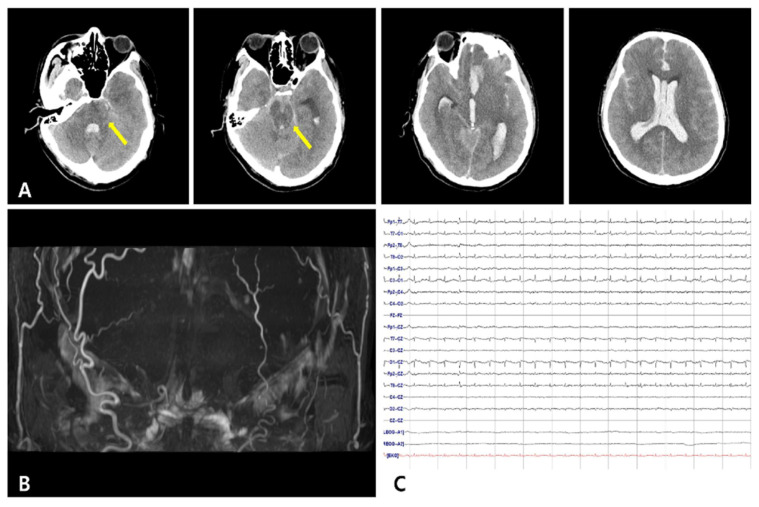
Radiologic findings and electroencephalography of the patient. (A) Brain computed tomography shows intracerebral hemorrhage in the left frontal lobe and massive intraventricular hemorrhage with subarachnoid hemorrhage including the basal cistern. Hypodense lesions in the brain stem were observed due to hypoxic brain damage (arrow). (B) Brain magnetic resonance angiography of the patient shows no cerebral blood flow. (C) There is no identifiable cerebral activity in any lead throughout the recording, even at 2 µV/mm sensitivity.
